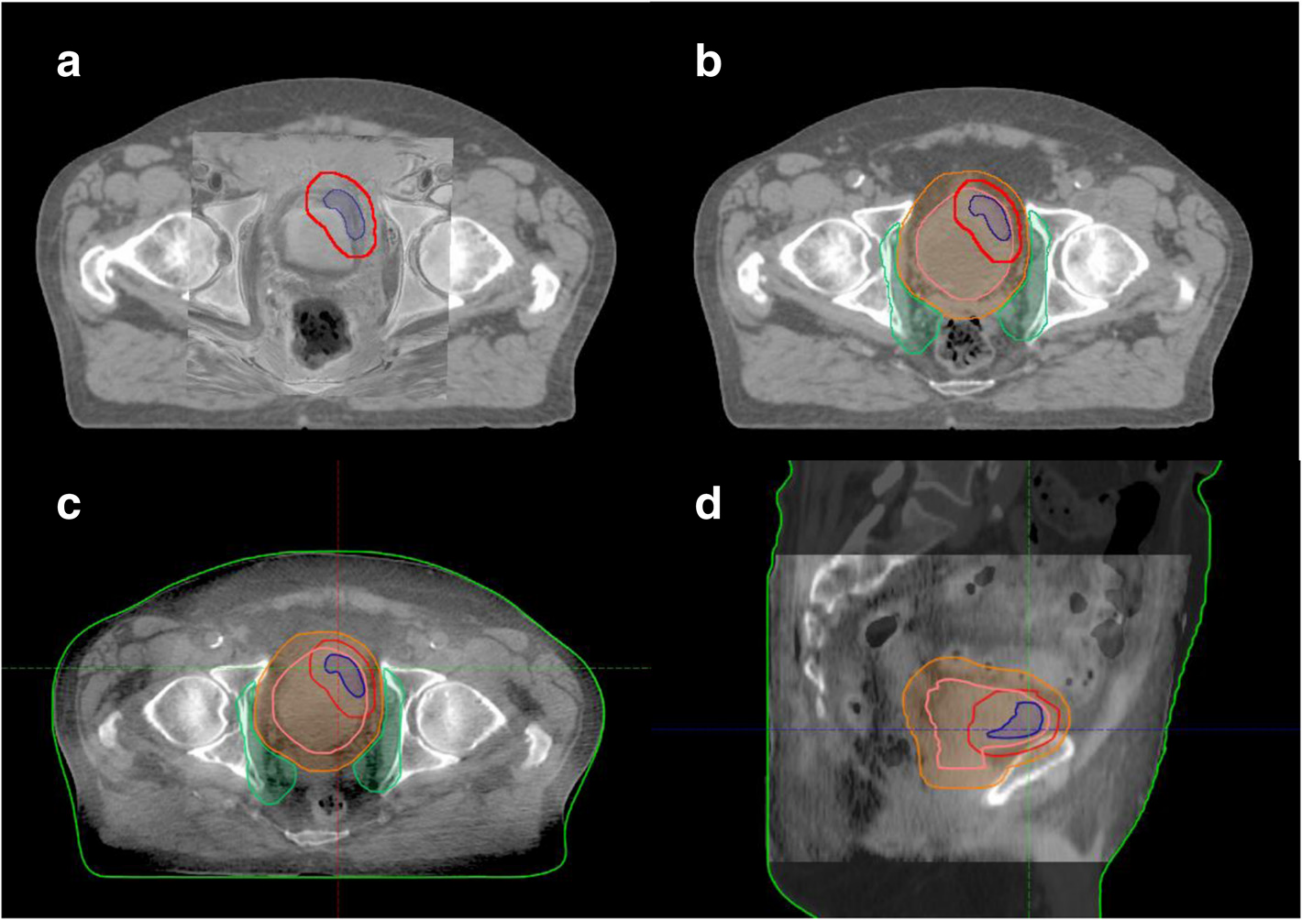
GTV delineation and soft tissue match to GTV on CBCT. Figs. a and b demonstrate the following contours as delineated on MR fusion (a) and planning CT (b): GTV – blue; High Risk PTV (HRPTV) – red; Bladder - pink; Intermediate Risk PTV (IRPTV) – purple and Low Risk PTV (LRPTV) – light brown. Figs. c and d show these contours overlaid on the cone beam CT to facilitate soft tissue match
